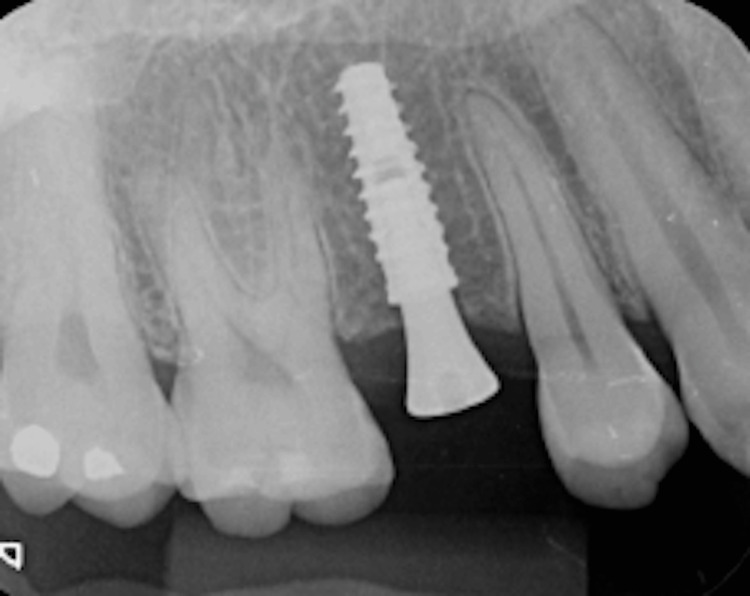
Final radiograph of implant 15 immediately after placement, with the healing abutment in place
